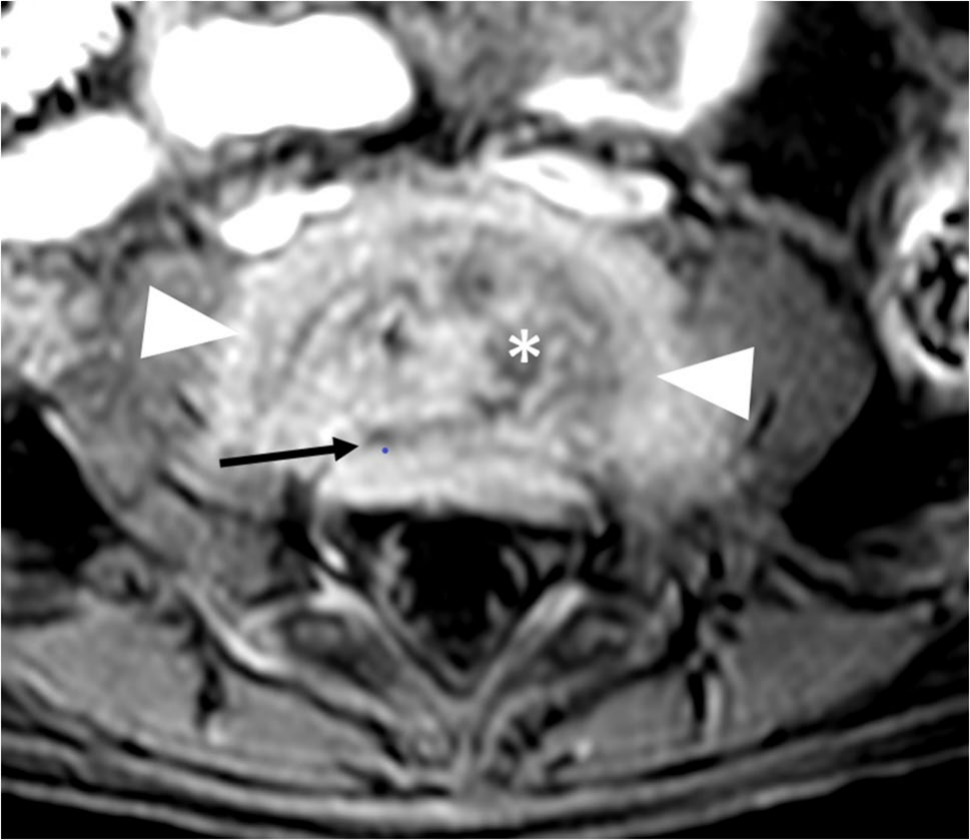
Magnetic resonance imaging of the lumbar spine in a 21-month-old girl with spondylodiscitis, performed 9 days after the ultrasound (Fig 1). Axial contrast-enhanced T1-weighted image at the level of the fourth and fifth lumbar intervertebral space demonstrates partial resolution of the epidural collection (*arrow*) and stable paravertebral enhancement (*arrowheads*). The disc still has a heterogeneous signal (*asterisk*)
